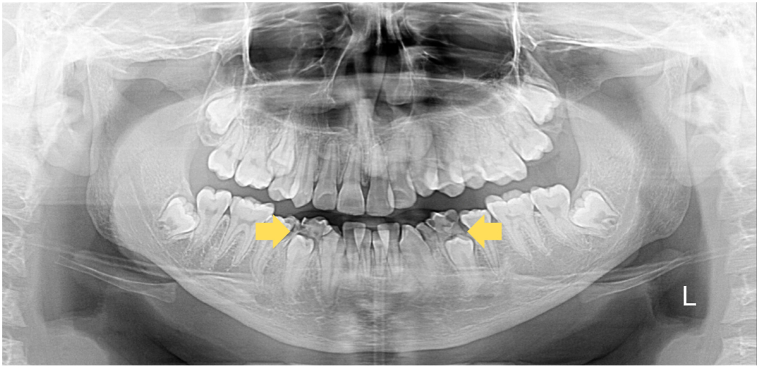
Dental panoramic X-ray showed retention of both mandibular first premolar primary teeth (*yellow arrows*).
